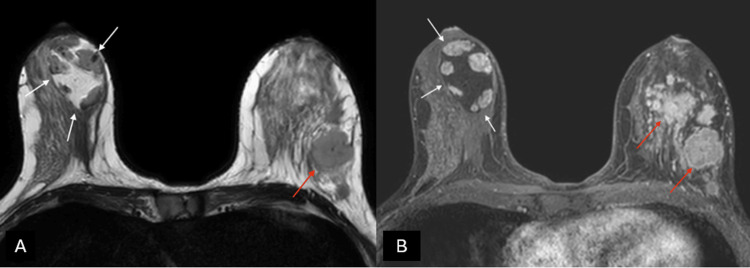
(A) Axial T2-weighted images and (B) T1-weighted fat-suppressed enhanced magnetic resonance imaging (MRI) A capsulated, large-sized hamartoma can be seen located in the outer quadrants of the right breast, exhibiting a signal identical to normal mammary gland tissue, creating a “breast within a breast” appearance, classified as BI-RADS 2 (white arrows). There are multiple masses in the contralateral breast with suspicious morphology, associated with a known invasive ductal carcinoma, classified as BI-RADS 6 (red arrows).
